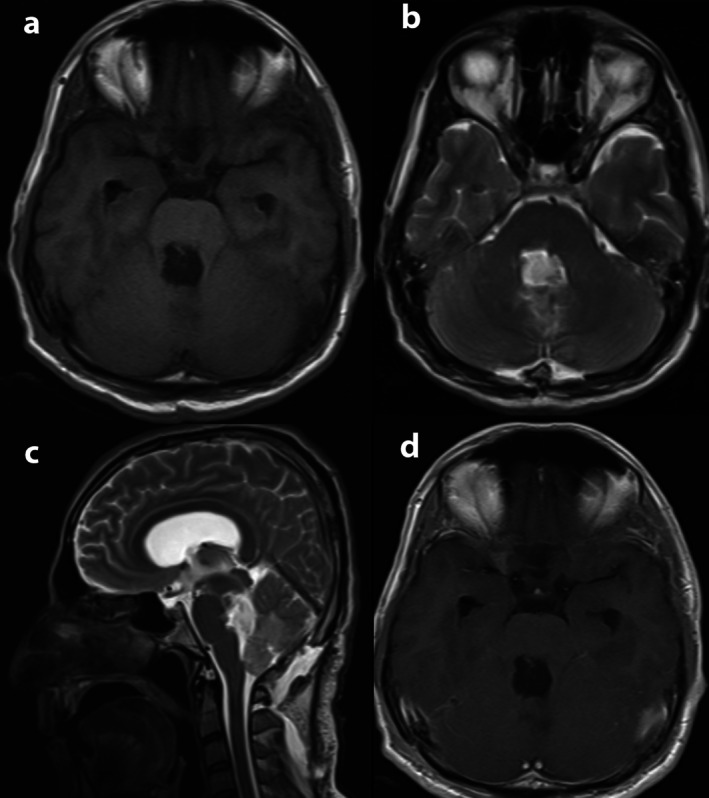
Postoperative MRI: (a) Axial T1W. (b) Axial T2W. (c) Sagittal T2W. (d) T1W with gadolinium.
